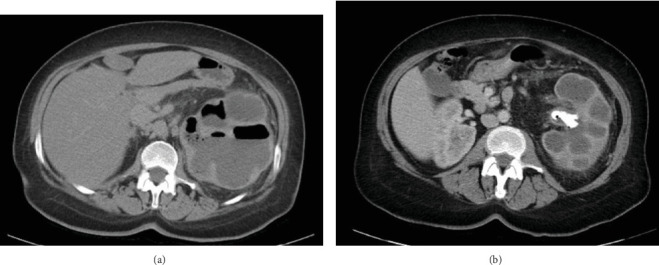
CT abdomen and pelvis with contrast axial views demonstrating severe hydronephrosis, dilated calices, and thin renal parenchyma of the left kidney. (a) Gas within the left renal collecting system in the calices. (b) Large stone within the left renal pelvis measuring 3.3 cm.
